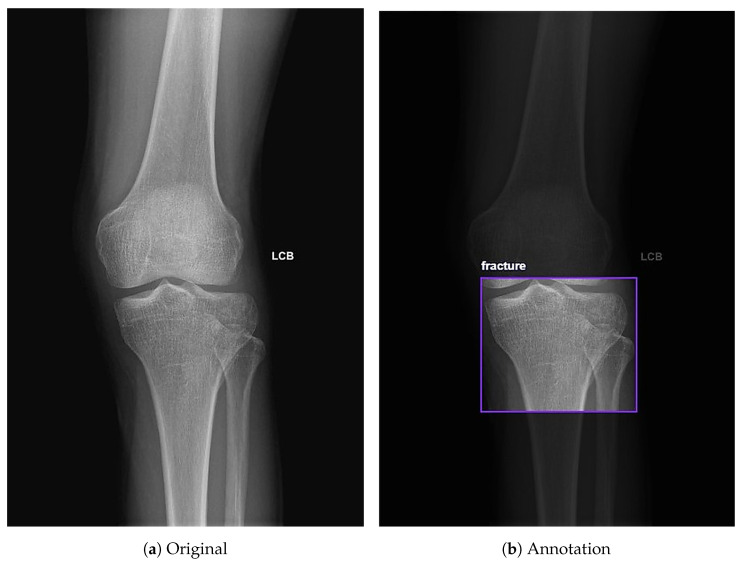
Annotation of knee X-rays using Roboflow. (a) Full-field AP view of the knee. (b) Example of an annotation on the tibial plateau labeled as fracture using Roboflow.
